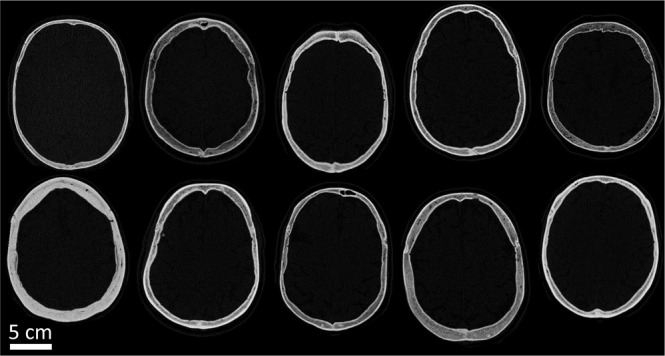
Computed tomography images of patient skulls demonstrate heterogeneity across patients. There is variability in size, thickness, shape, and proportion of cortical and trabecular bone within and across patients.
